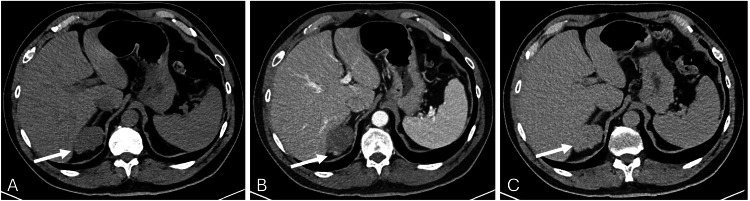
CT scan of the abdomen with and without contrast (adrenal protocol) (A) Non-contrast CT image demonstrates a lobulated hypodense lesion noted posterosuperior to the right adrenal gland, closely abutting the adjacent segment 7 and right adrenal gland (white arrow). (B) Post-contrast CT image in the porto-venous phase shows peripheral tiny nodular enhancement (white arrow). (C) A 15-minute delayed post-contrast image demonstrates homogeneous enhancement of the lesion (white arrow), consistent with centripetal contrast filling.
